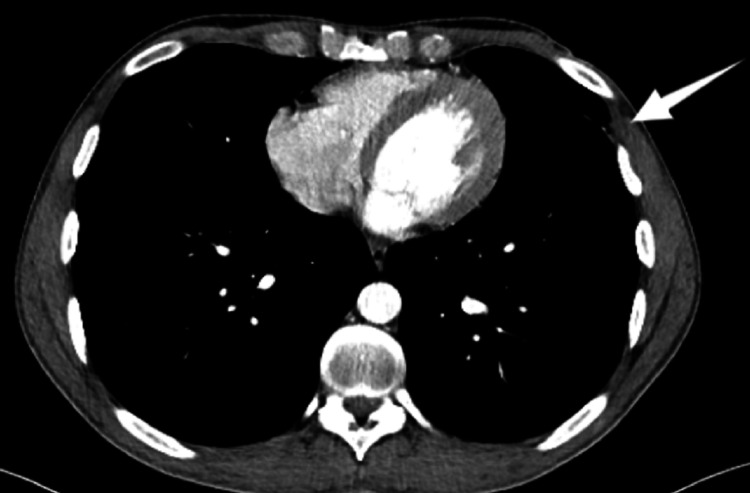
Enhanced axial slice of the chest CT in the mediastinal window at three months post-treatment demonstrating complete resolution of the chest wall collection and the presence of a pleural cicatricial band at the site of the previously observed fistula (arrow).
